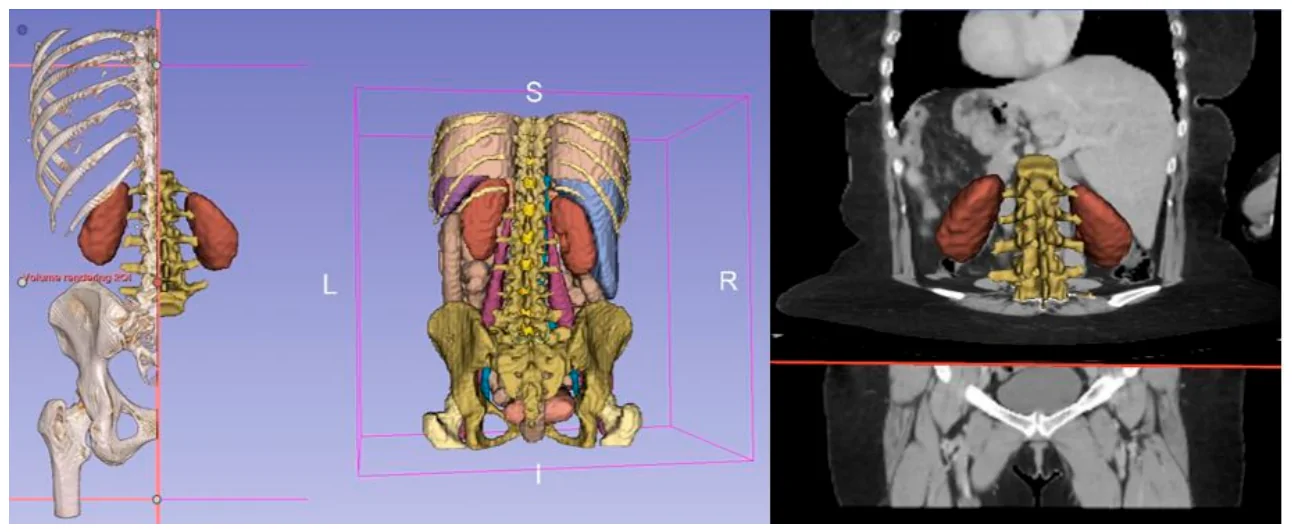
Representative three-dimensional reconstruction of the automatically segmented kidneys generated using the Total Segmentator framework within 3D Slicer. The segmented kidneys are displayed together with the vertebral column to provide anatomical orientation, while the corresponding coronal CT image demonstrates the anatomical localization of the segmented structures before morphometric analysis (L: left, R: right, S: superior).
